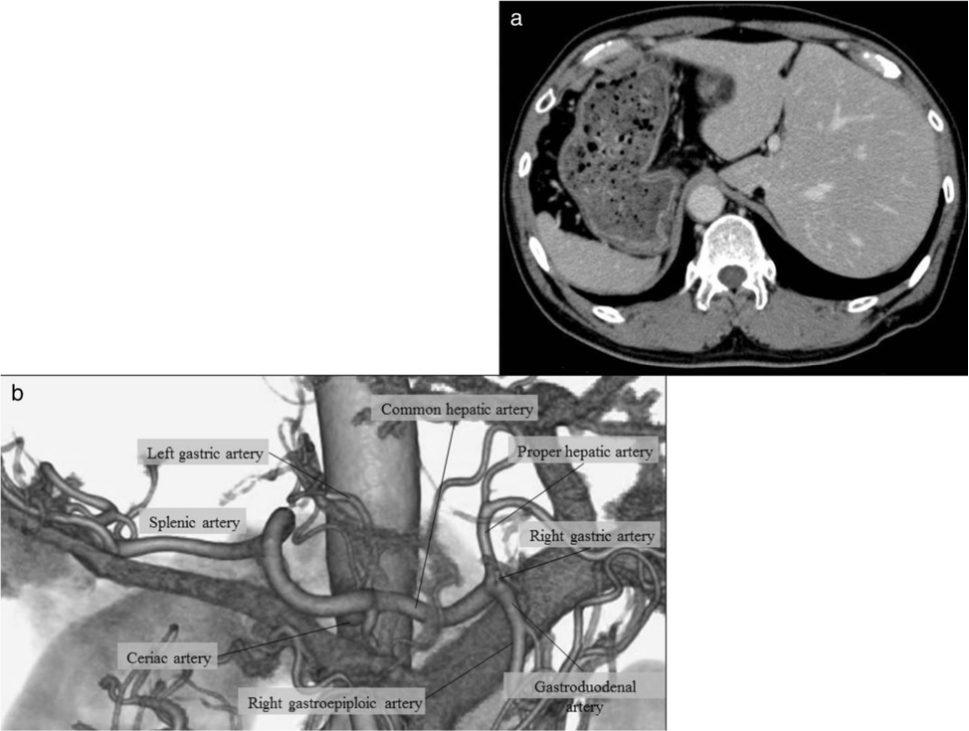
a Enhanced abdominal CT showed transposition of the abdominal organs and identified no metastasis to the lymph nodes or to the distant organs. b Three-dimensional reconstruction image of CT angiography showed no vascular anomalies
